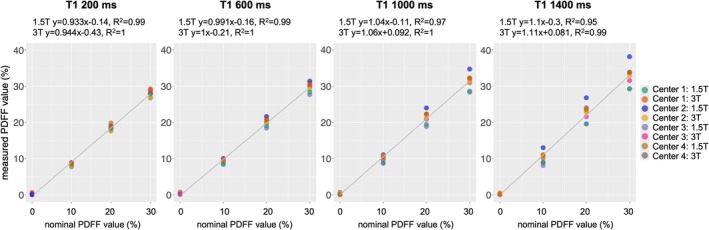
Linear regression shows good agreement in PDFF values across centers and platforms, with higher variability in the presence of high fat and T_1_. Depicted are reference (nominal) and measured CSE‐MRI PDFF values across 4 centers and 8 different systems. The columns represent vials with nominal water‐specific T_1_ of 200 ms, 660 ms, 1000 ms and 1400 ms, respectively. The regression equations predict measured PDFF (y‐axis) based on nominal PDFF (x‐axis). Vendors: Center 1, GE HealthCare; Center 2, Philips Healthcare; Center 3, Siemens Healthineers; Center 4, Canon Medical Systems. Abbreviations: PDFF, proton density fat fraction; CSE‐MRI, chemical shift‐encoded MRI.
